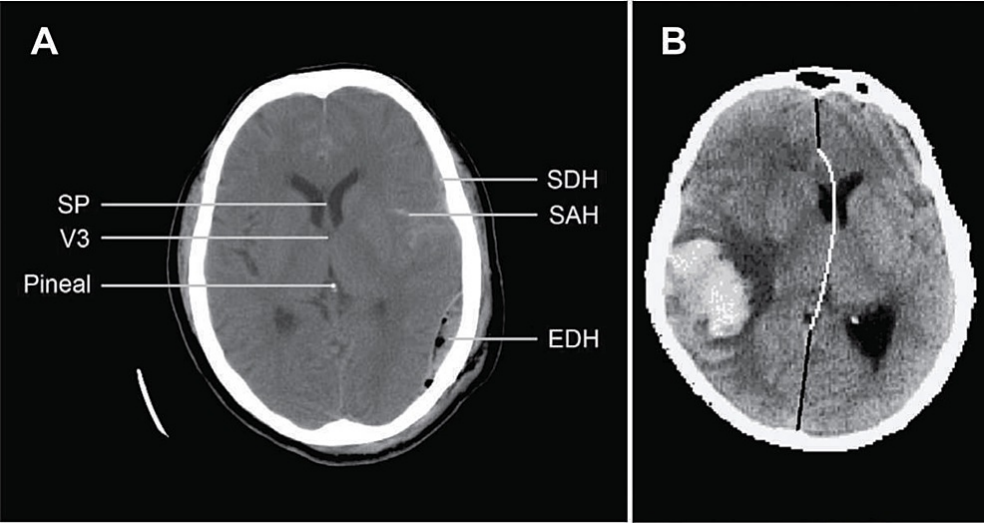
(A) is a CT image of a traumatic brain injury with various anatomical landmarks, which is used to measure the midline shift (MLS) along with different intracranial hemorrhages. (B) is suggestive of MLS due to intracerebral hematoma (ICH) that compresses the brain. SP: septum pellucidum; V3: third ventricle (only the most rostral part shown); SDH: subdural hematoma; SAH: subarachnoid hemorrhage; EDH: epidural hematoma
